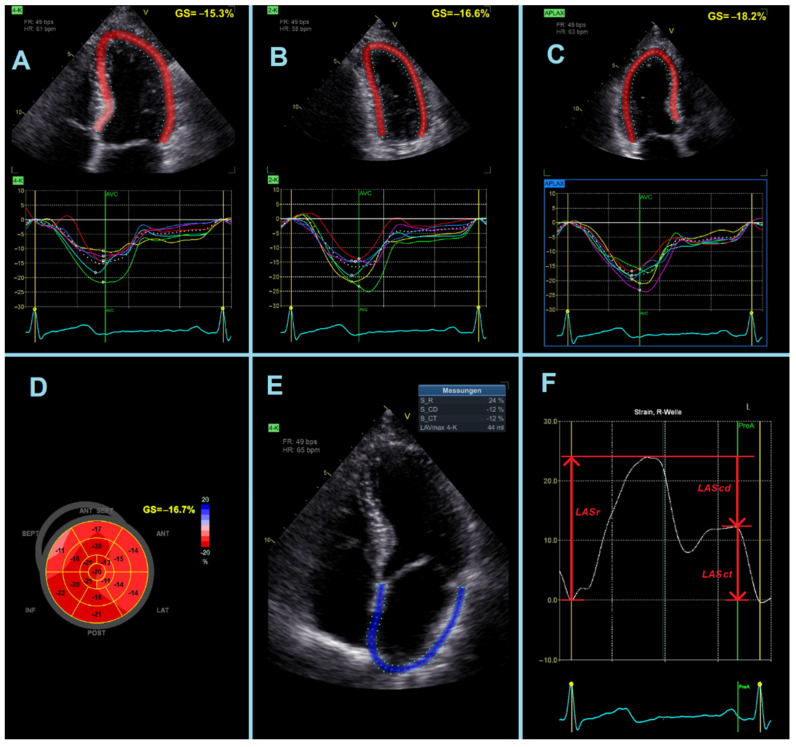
Speckle tracking echocardiography with left ventricular global longitudinal strain (LV-GLS) assessment (A) apical 4-chamber view; (B) apical 2-chamber view; (C) apical 3-chamber view; (D) Bull’s-eye plot), and left atrial strain assessment (LAS) in apical 4-chamber view (E,F).
